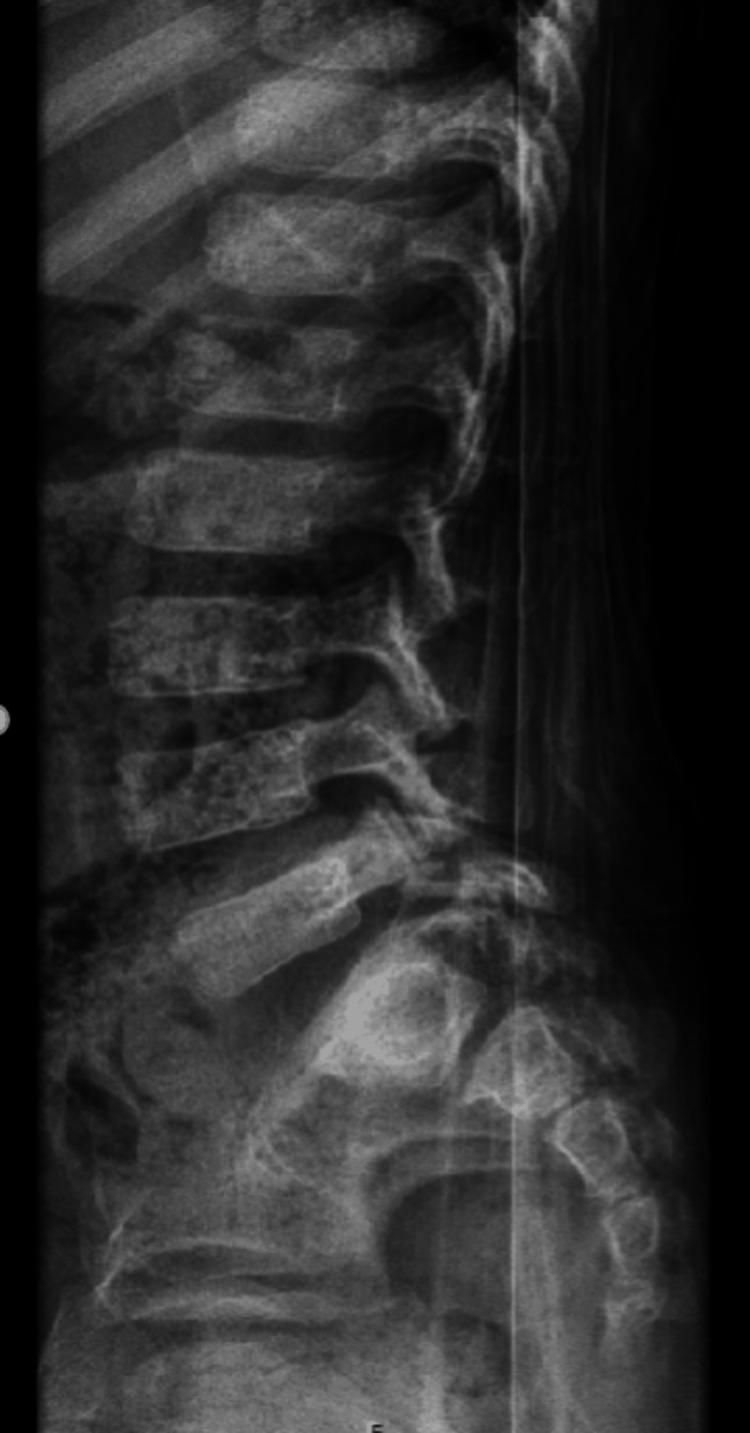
Radiograph showing suspected spondylolisthesis of L5-S1
